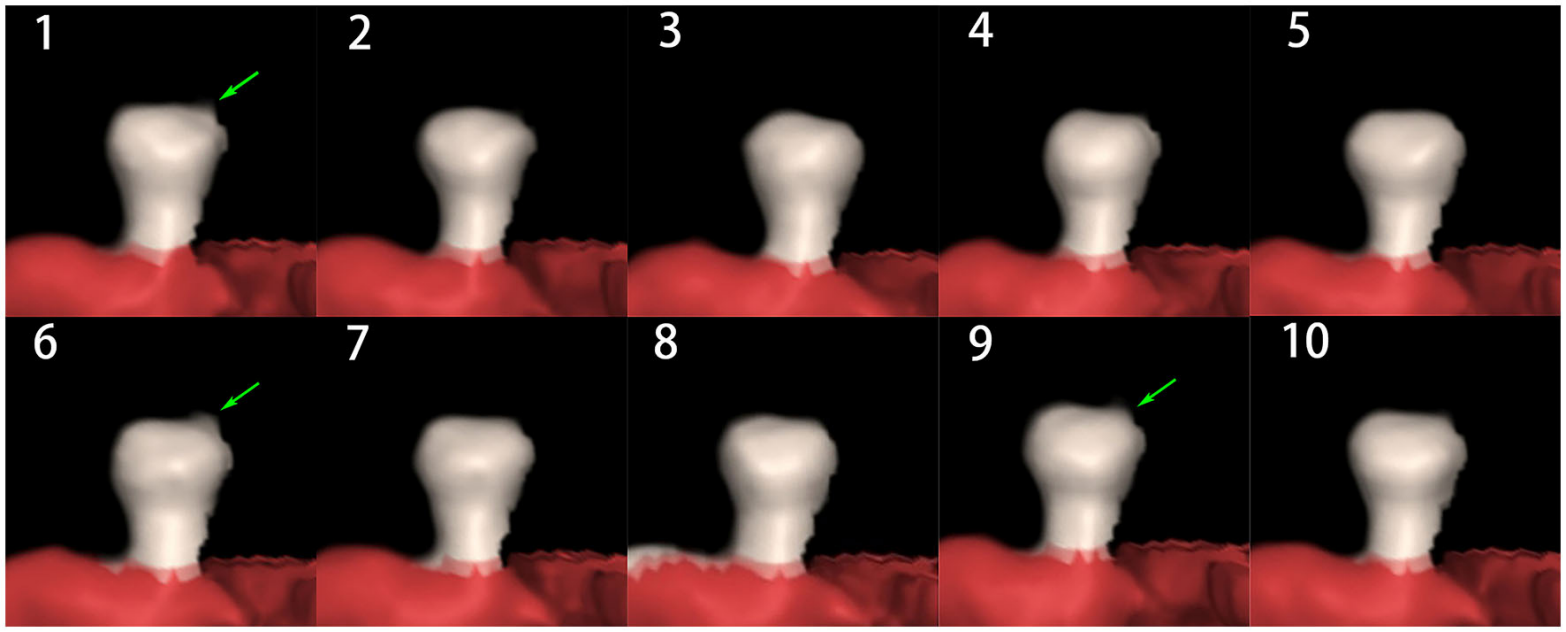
4D-CTA images in one cardiac cycle showing increased volume change (green arrow) in a 65-year-old woman with a ruptured aneurysm.
